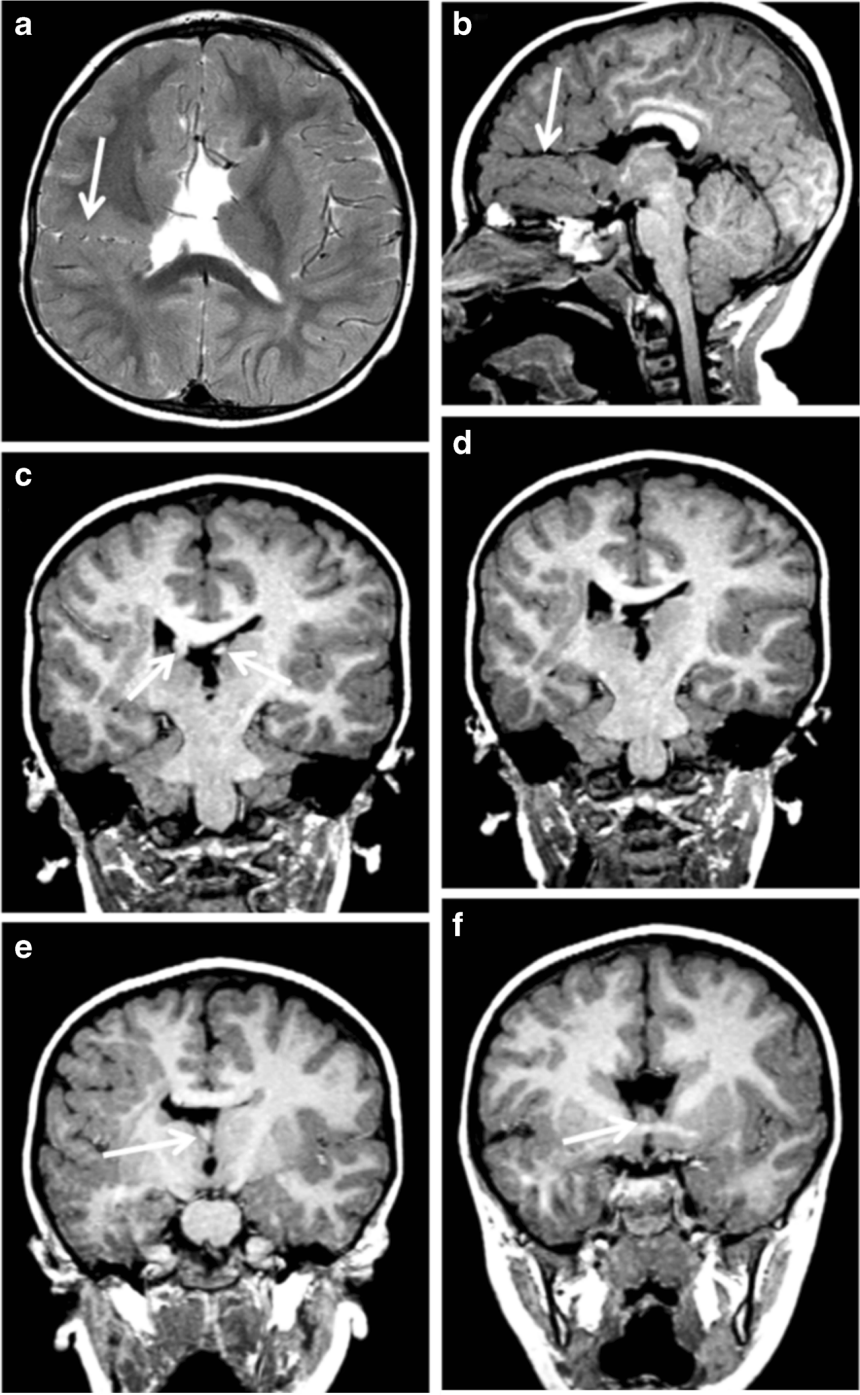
Two regions of schizencephaly (type 2) in the same hemisphere. MR images from a 2-year-old child with hemiparietic (left side of body) cerebral palsy. Axial T2-weighted image shows schizencephaly (type 2) related to the right paracentral lobule (arrowed on a) whilst the right parasagittal image from a T1 volume acquisition shows a further area of schizencephaly (type 2) in the inferior frontal gyrus (arrowed on b). The anterior part of the corpus callosum is absent and there is a low position of cerebellar tonsils. Coronal reconstructions from the T1 volume data from posterior to anterior (c–f) show an aberrant course of the fornices and distorted, thickened septum pellucidum (arrowed on c, e and f)
